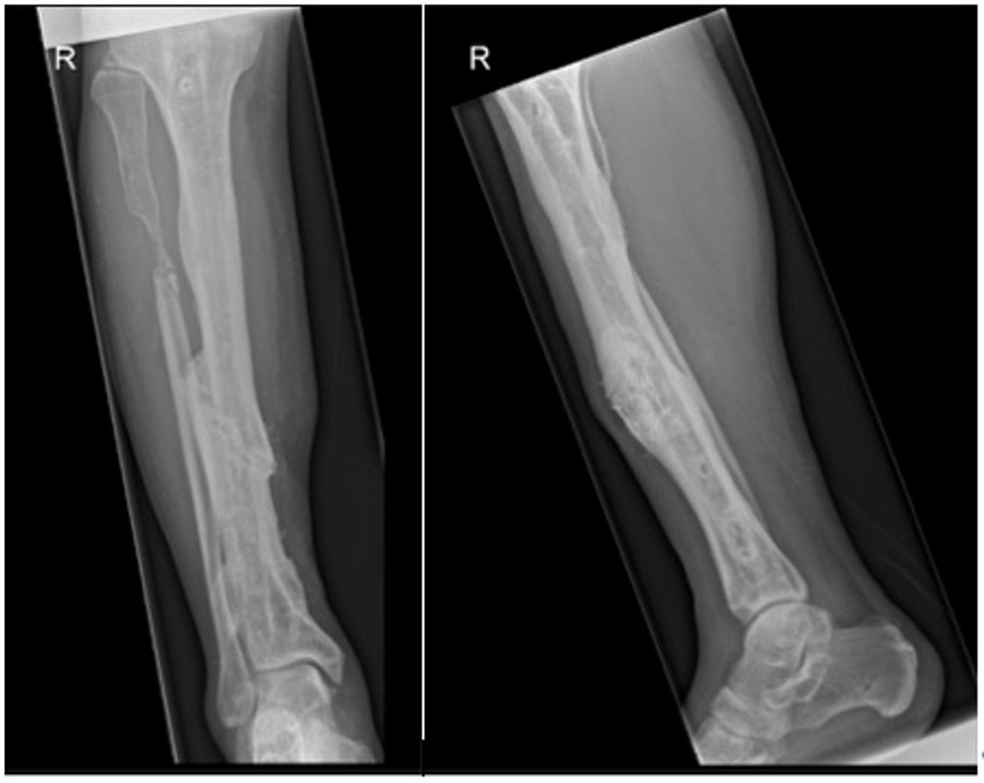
X-ray images showing a non-union of the right tibia
